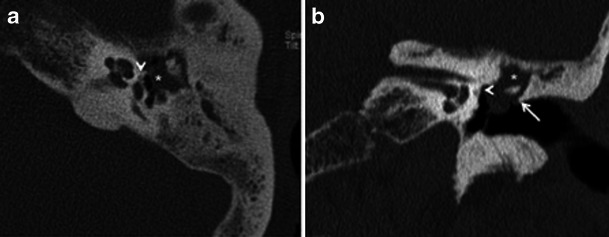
Axial (a) and coronal (b) HRCT images of the left temporal bone in an adult patient with left-sided CHL and previous history of left-sided otitis media. The soft-tissue density noted in the attic (*asterisk*) causing blunting of the scutum (*arrow*) is in favour of a cholesteatoma. In addition, a tiny hypodense fenestral otosclerotic focus (*arrowheads*) is noted anterior to the oval window
